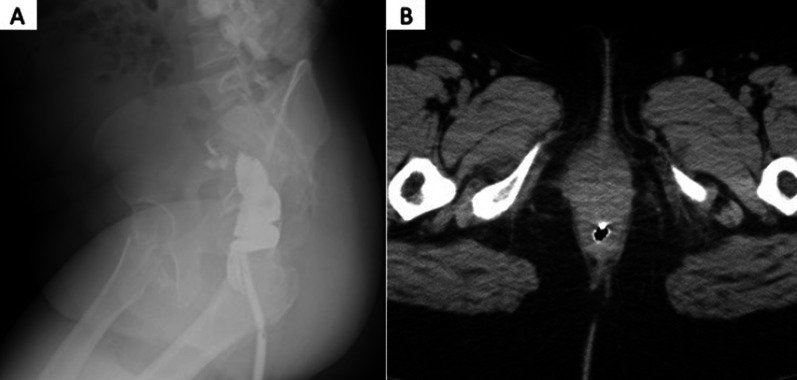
Gastrografin enema (A) using a water-soluble contrast medium through the transanal decompression tube, followed by a plain computed tomography (B)
